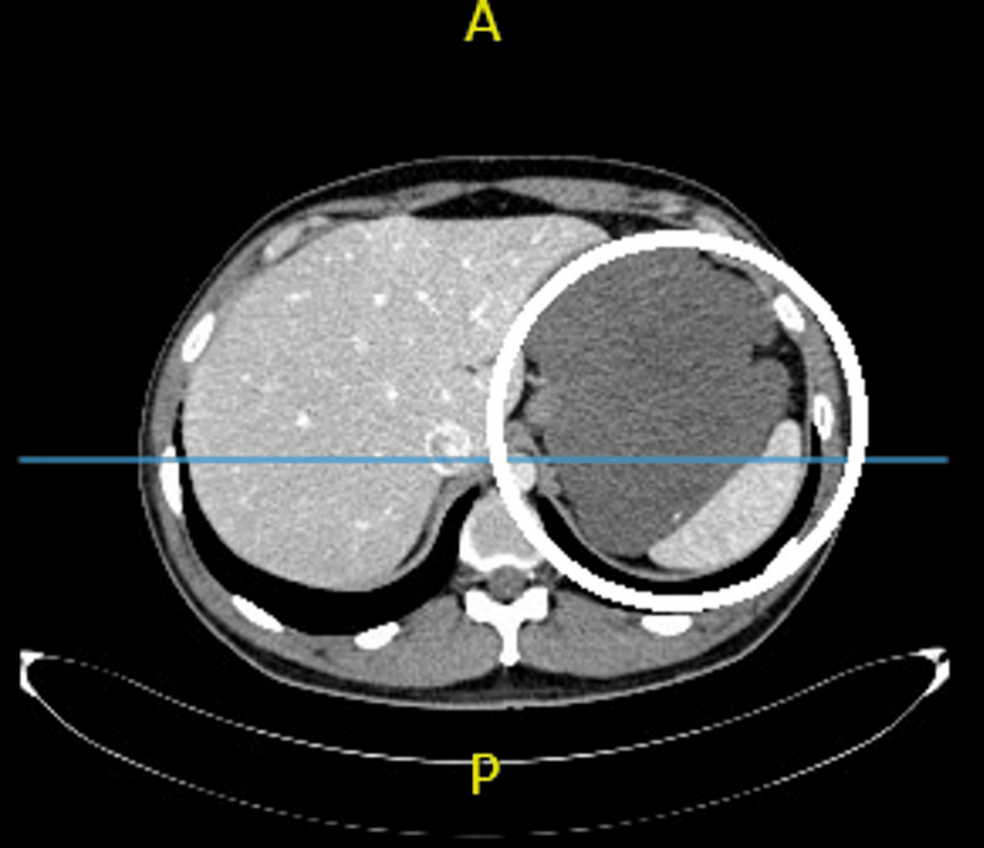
Abdominal CT images after neoadjuvant therapy with imatinib demonstrating a significant size regression and decreased enhancement of the giant intra-abdominal necrotic mass. The mass now measured 17 × 14 × 21 cm.
